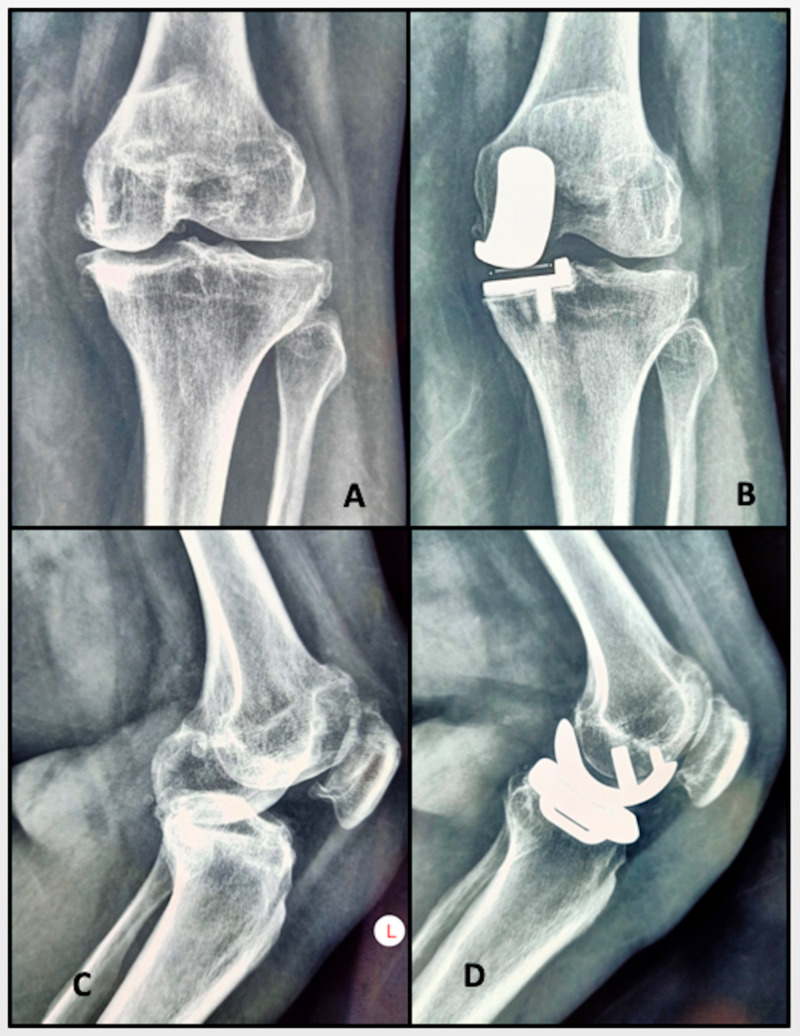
Preoperative and postoperative radiographs (A) Preoperative AP radiograph of the left knee showing medial compartmental osteoarthritis. (B) Postoperative AP radiograph of the left knee showing a well-fixed UKR prosthesis. (C) Preoperative lateral radiograph of the left knee. (D) Postoperative lateral radiograph of the left knee with the implant in situ. AP, anteroposterior; UKR, unicondylar knee replacement
